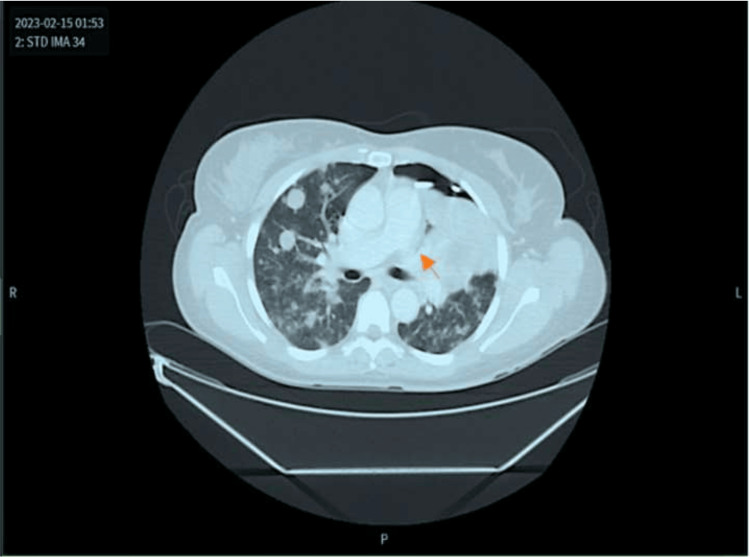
Axial-plane CT with coronal reconstruction (orange arrow) revealed complete left upper lobe collapse.
